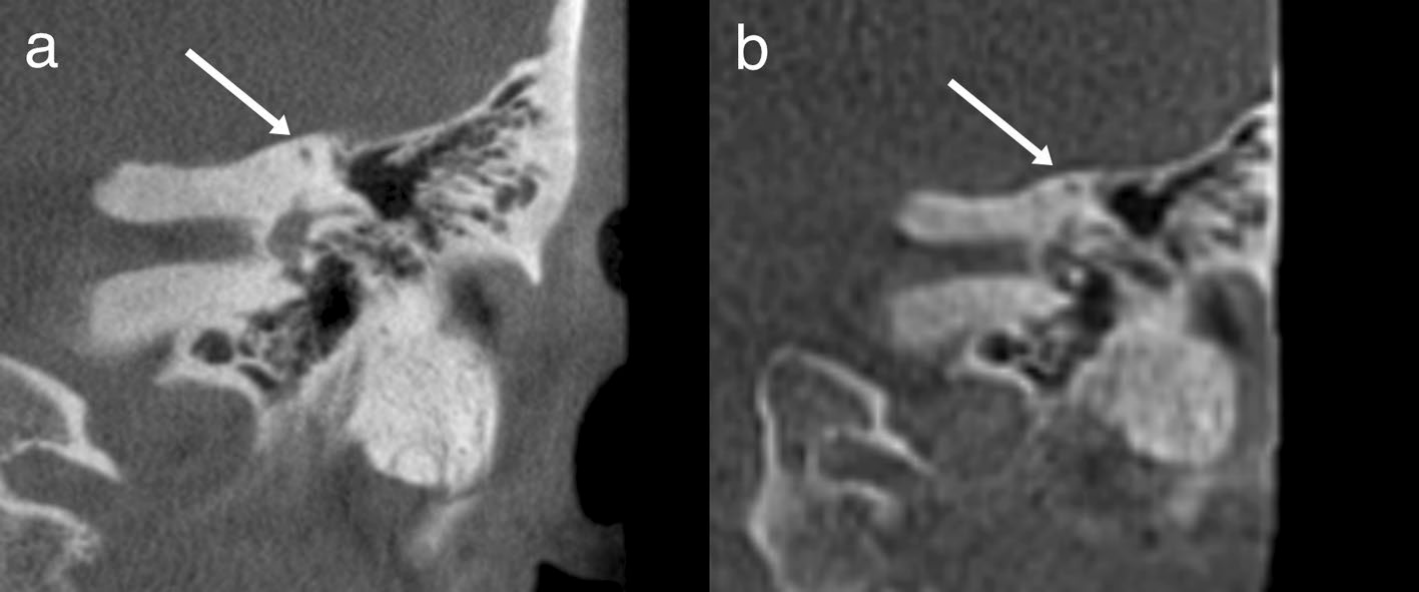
Example images in the coronal plane taken from a single patient, one with HR CBCT (a) and one with ULD CBCT (b). The bony coverage of the superior semi-circular canal is visible
